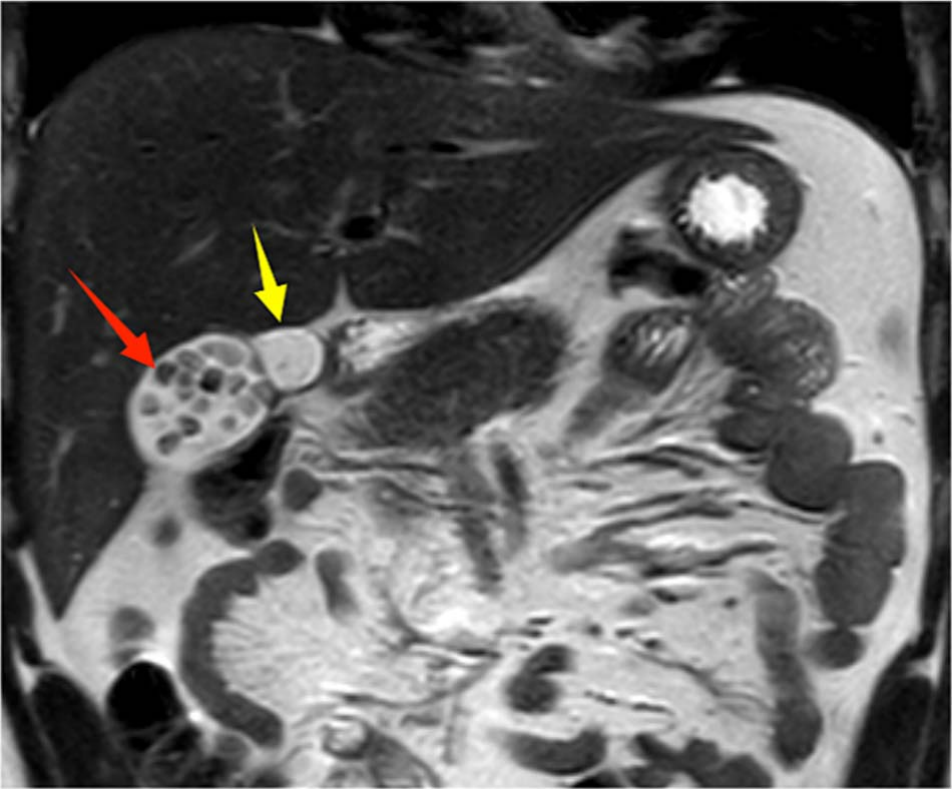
Upper abdominal MRI coronal view demonstrates a double gallbladder variation. The two arrows indicate the respective gallbladders, the lateral one of which contains multiple gallstones.
